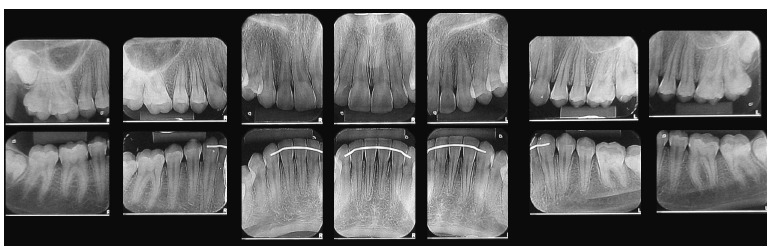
Final periapical radiographs.
